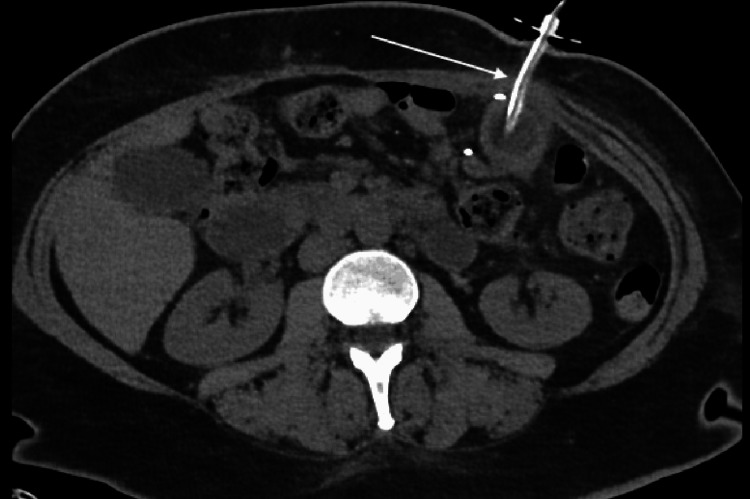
Post-procedural axial CT following percutaneous gastrostomy Post-procedural axial CT image demonstrating decompression of the excluded stomach after gastrostomy tube placement (white arrow).
